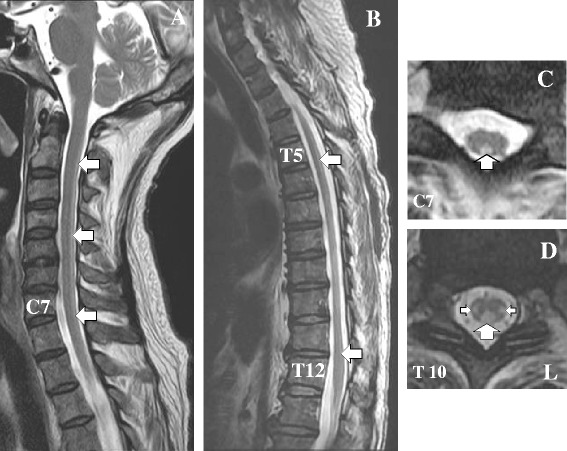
Conventional T2-weighted magnetic resonance imaging of the cervical and thoracic spinal cord after 6 weeks of intrathecal administration of methotrexate. a Sagittal image shows signal hyperintensity (arrows) in the cervical spine from C2 to C7. b Sagittal image shows signal hyperintensity (arrows) in the thoracic spine from Th4 to Th11. c Coronal image shows signal hyperintensity in the posterior column at the C7 level (arrow). d Coronal image shows signal hyperintensity in bilateral dorsal columns at the Th10 level (arrows)
